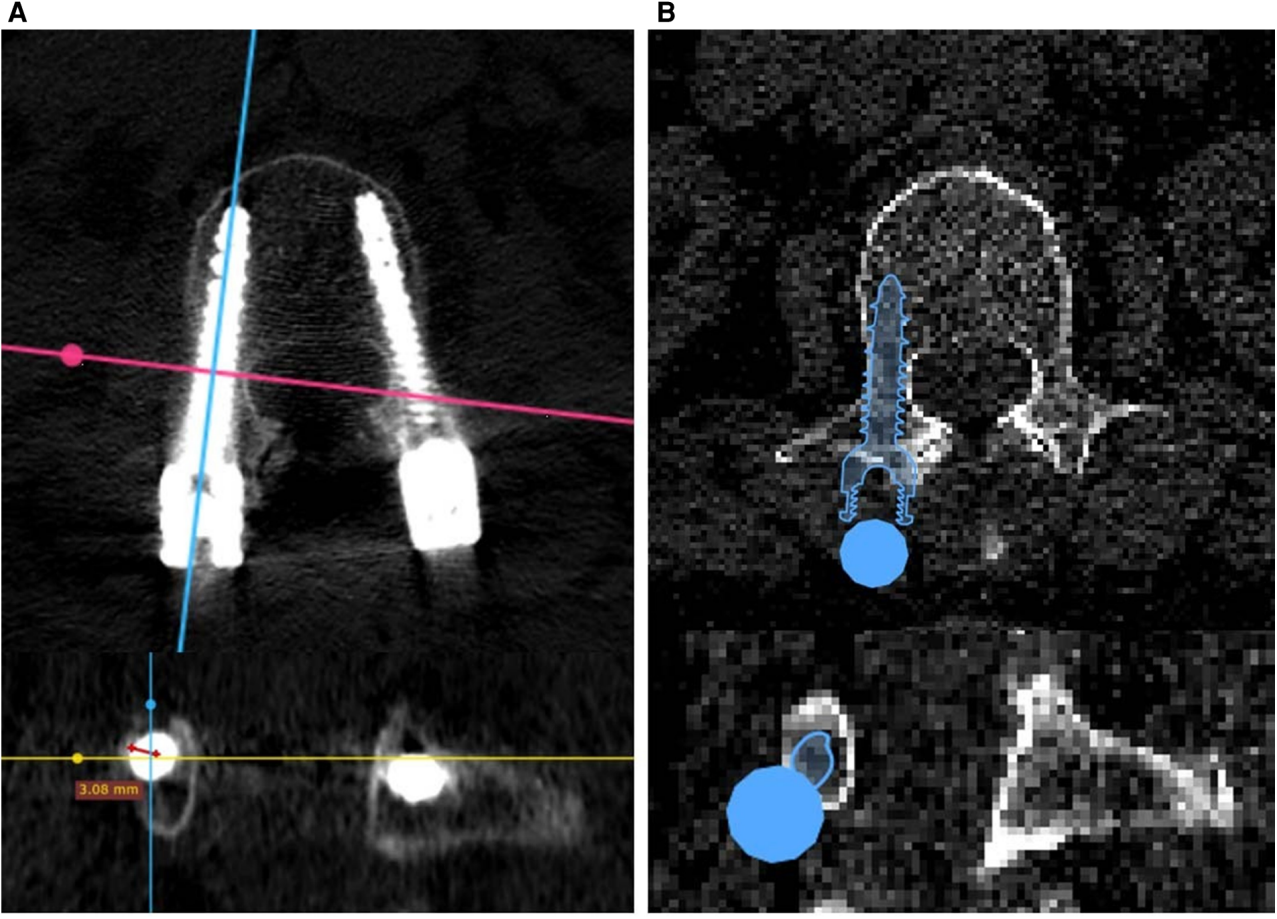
Gertzbein–Robbins evaluation of (A) freehand surgeries based on postoperative CT images and (B) AI planning results based on preoperative CT images.
